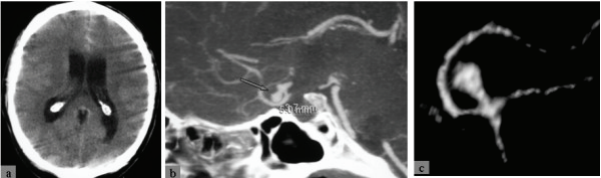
Brain CT scan on admission (A). CT angiogram revealed an anterior communicating aneurysm measuring 3.7 x 5 mm (B arrow, C).
